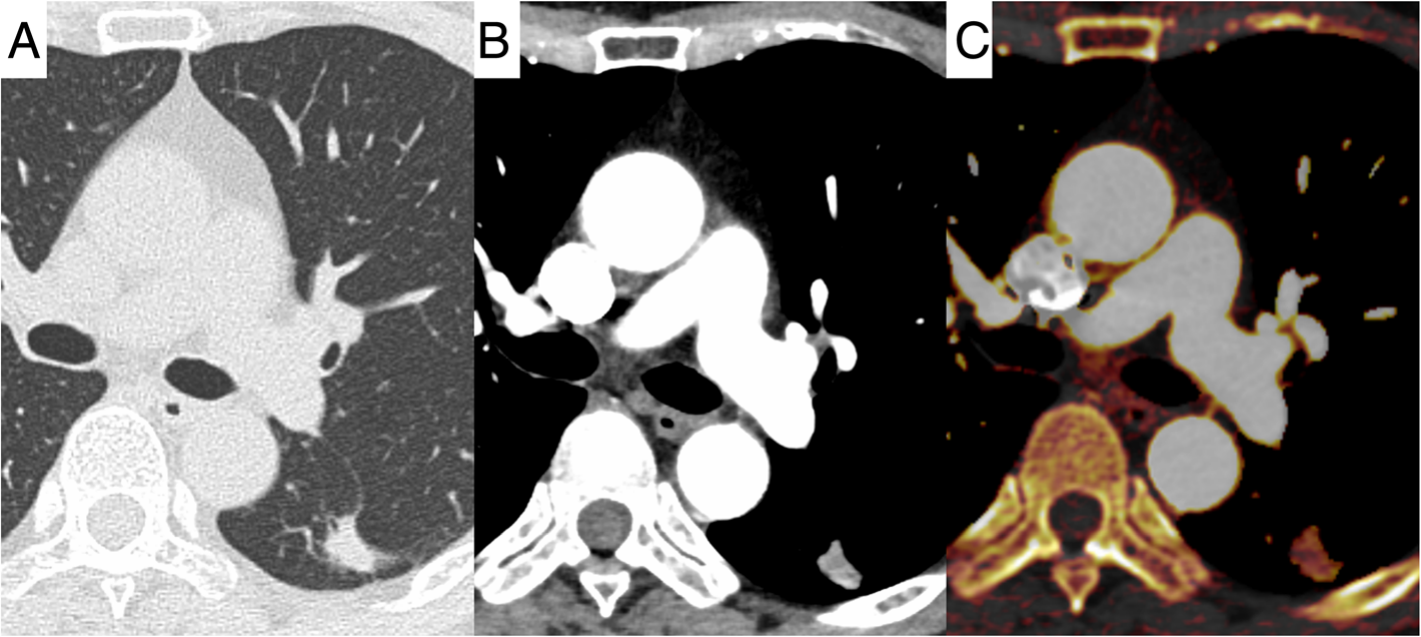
A 66-year-old female patient. A well-differentiated (G1) adenocarcinoma is observed in the left lower lobe. Lymphatic permeation, vascular invasion, and pleural involvement were all negative. a Lung window setting. b Mediastinal window setting. c Iodine-enhanced image of contrast-enhanced CT. The 3D-IRA is 47 HU, and the corrected 3D-IRA is 8.13
